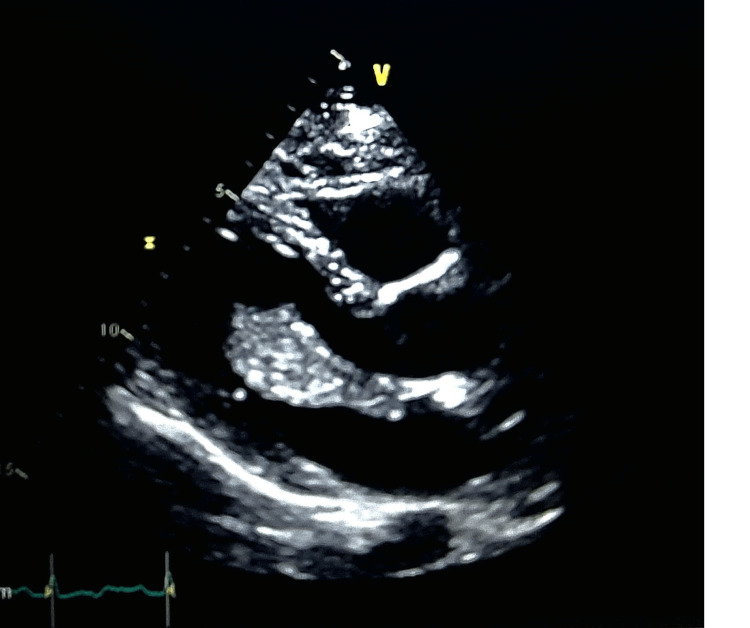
Echocardiogram showing a myxoma in the left atrium. Echocardiogram demonstrating a mass noted in the left atrium. The mass measures approximately 35 mm by 35 mm. The left atrial mass is suggestive of a myxoma.
